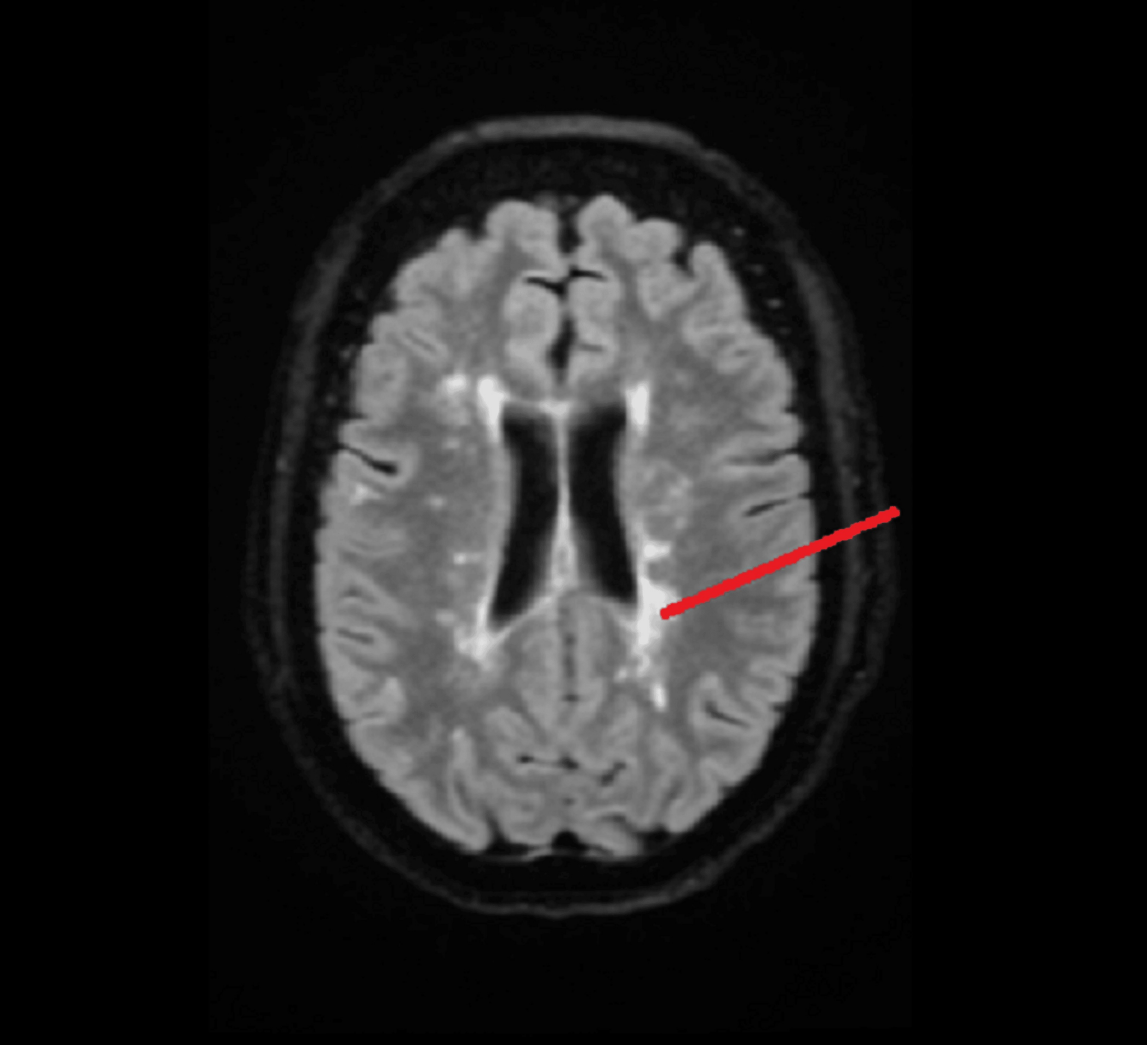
Transverse view of brain MRI The red line points to classic Dawson’s fingers on the brain MRI.
